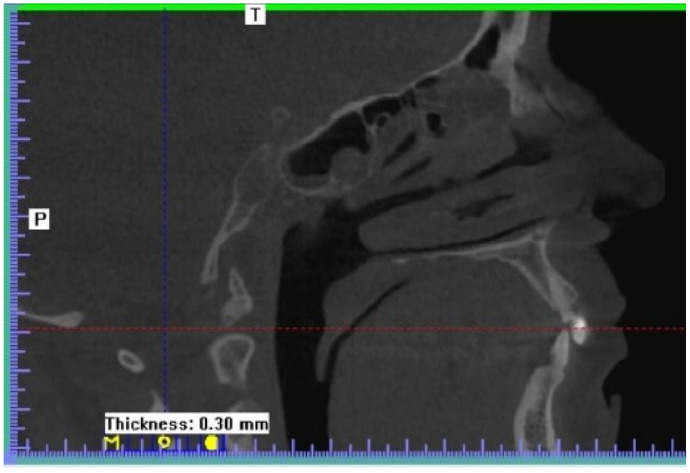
Mucosal thickening in ethmoid cells and at the bottom of the sphenoid sinus—CBCT scan—sagittal view.
